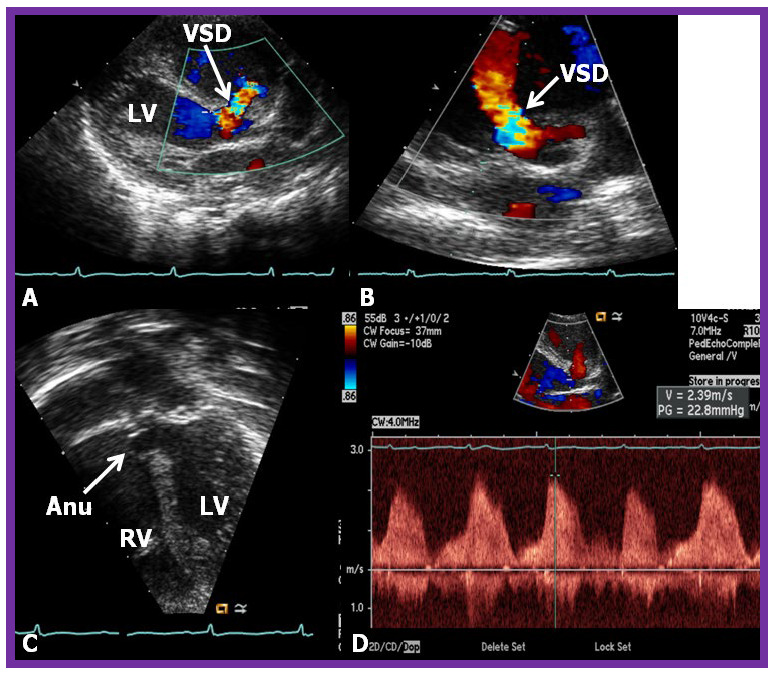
Comprehensive echo-doppler analysis of a perimembranous VSD.
